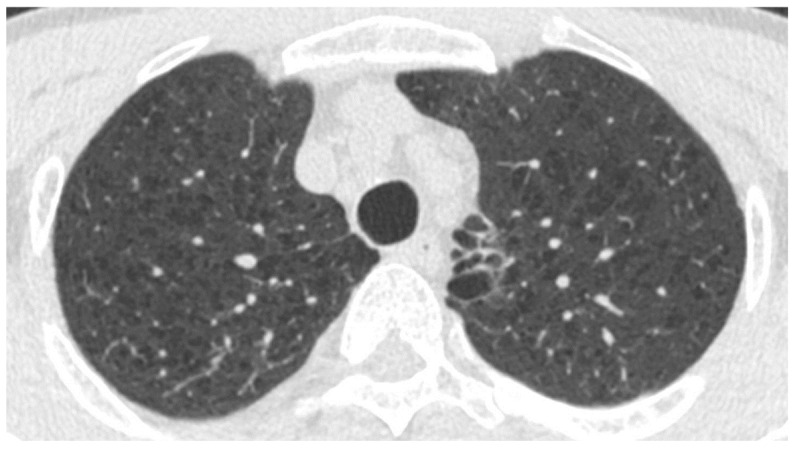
An axial low-dose computed tomograph of a 63-year-old male demonstrating centrilobular and paraseptal emphysema predominantly in the bilateral upper lobes of the lung.
